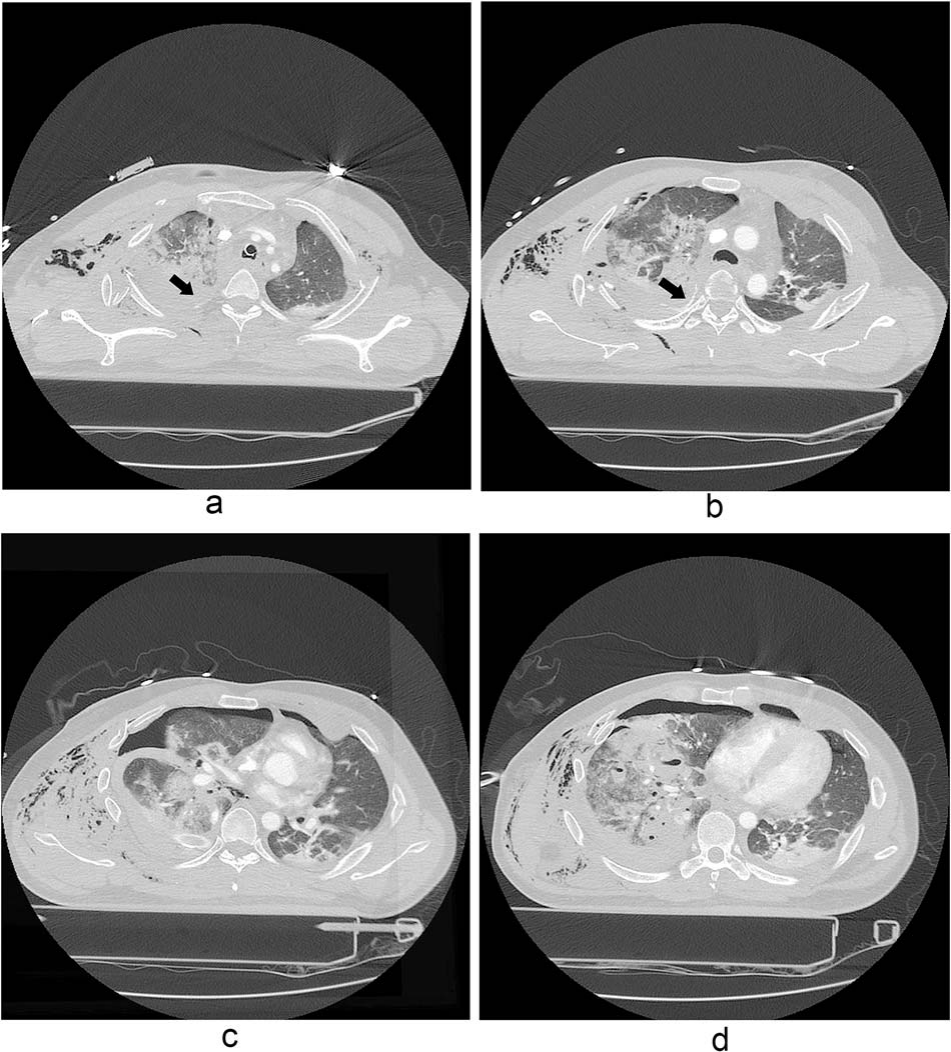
A contrast-enhanced chest CT scan after blunt chest trauma. Chest radiograph showed right hemopneumothorax and left hydropneumothorax. Multifocal extensive hemorrhage/pneumatocele formation was visible on the right side along with combined active bleeding (arrows) in the right lung.
